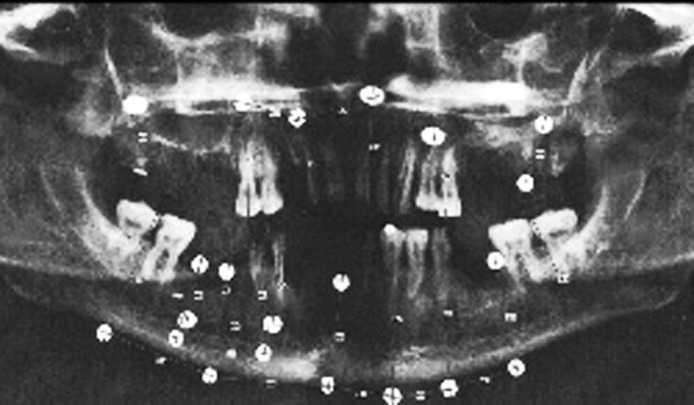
Skull number 1 on normal head position. Panoramic radiograph shows lead markers in different regions of the jaws
